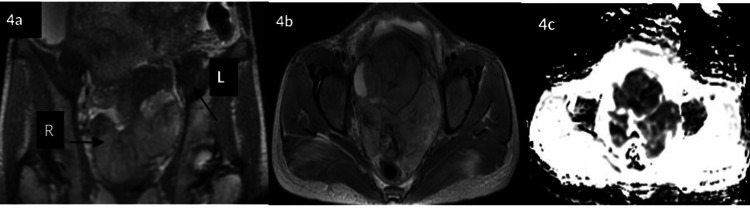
MRI highlighting bulky ovaries The coronal (a) and axial (b) T2 sequence shows bilateral bulky ovaries (black arrows) and blooming foci on axial GRE with mild homogenous enhancement on post-contrast T1 sequence. Small areas of diffusion restriction are visualized in the bilateral ovaries on the diffusion weighted imaging (DWI)-ADC sequence (c), suggesting metastatic involvement. ADC: Apparent diffusion coefficient
